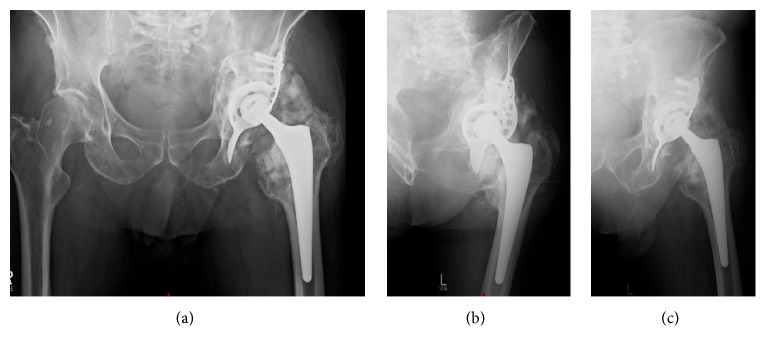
Follow-up X-ray (a.p., obturator and ala-view) one year postoperatively.
